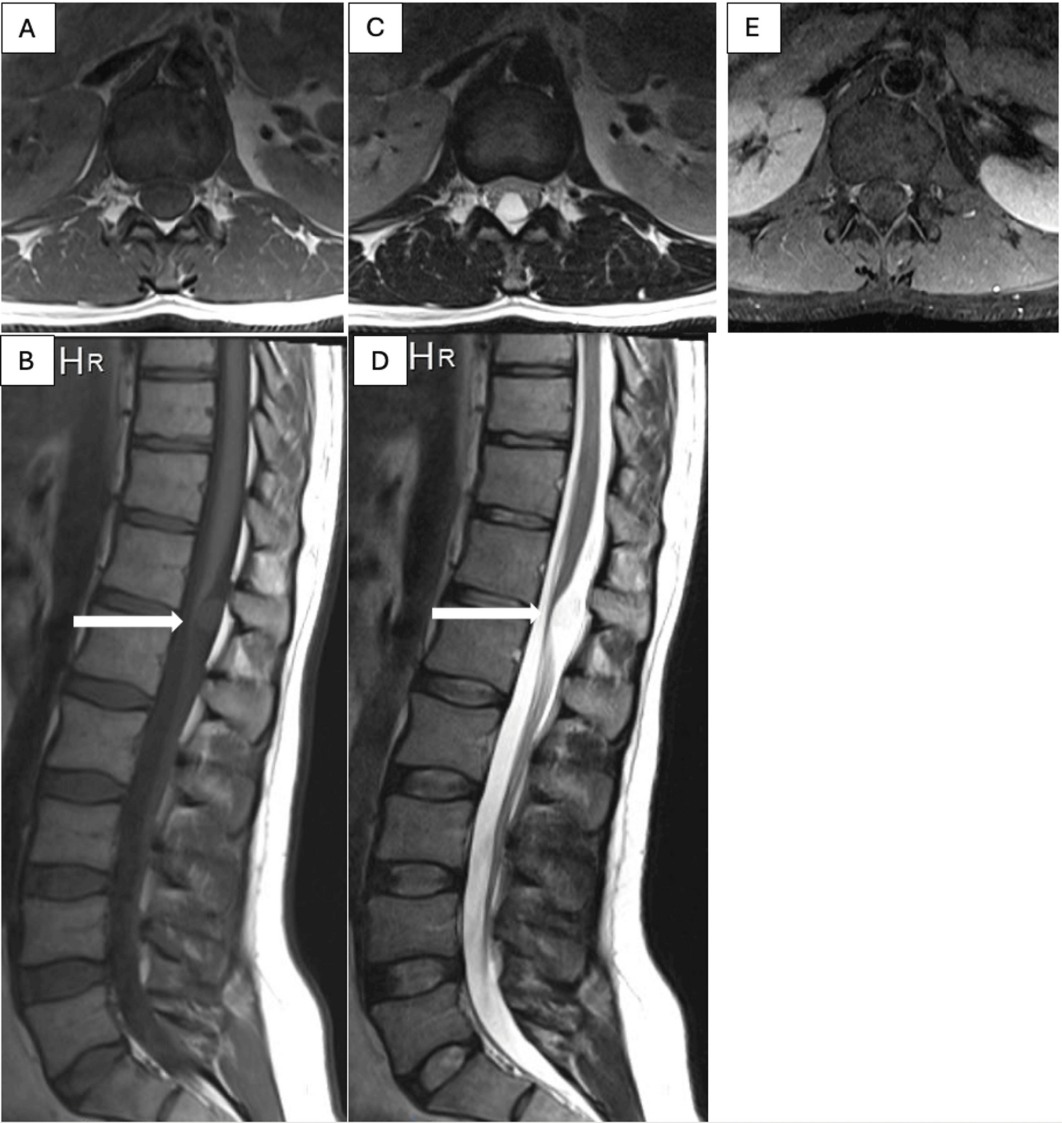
Magnetic resonance imaging (MRI) of the lumbar spine without and with contrast. MRI showing a 1.1 x 1.3 cm well-defined intradural extra-medullary lesion at the level of T12-L1, with isointense signal intensity on axial and sagittal T1WI (A, B), high signal intensity on axial and sagittal T2WI (C, D), and no significant enhancement post-contrast (E). T1WI: T1-weighted images, T2WI: T2-weighted images
